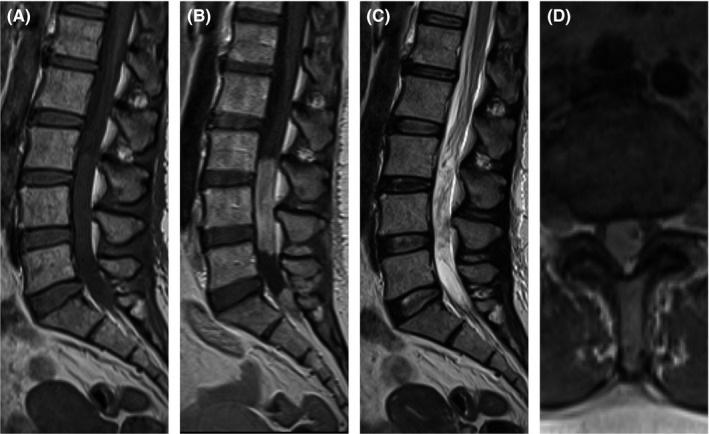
MRI spine, selected images. Sagittal T1 pre‐(A) and post‐(B) contrast, T2 weighted (C) and axial T1 postcontrast (D) images demonstrate T1‐isointense, T2‐hyperintense intradural and extramedullary lesion centered on the filum terminale which enhances avidly following contrast administration. Radiological appearances are compatible with a myxopapillary ependymoma
